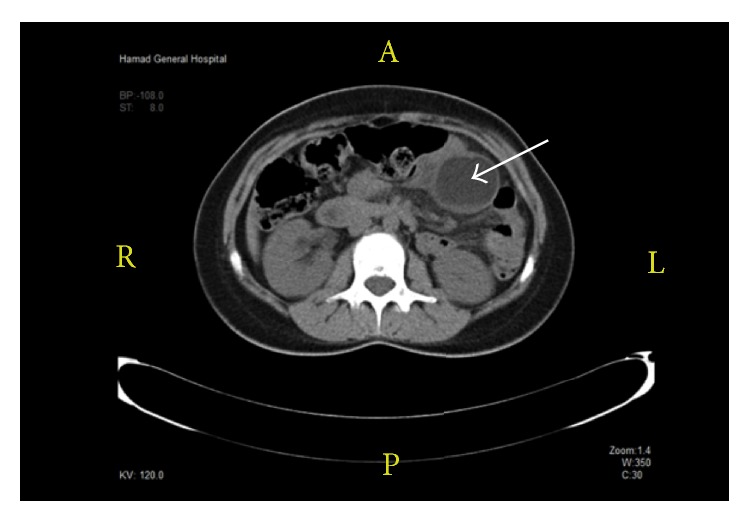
Case 1. Computed tomography scan showing mesenteric cyst (white arrow).
